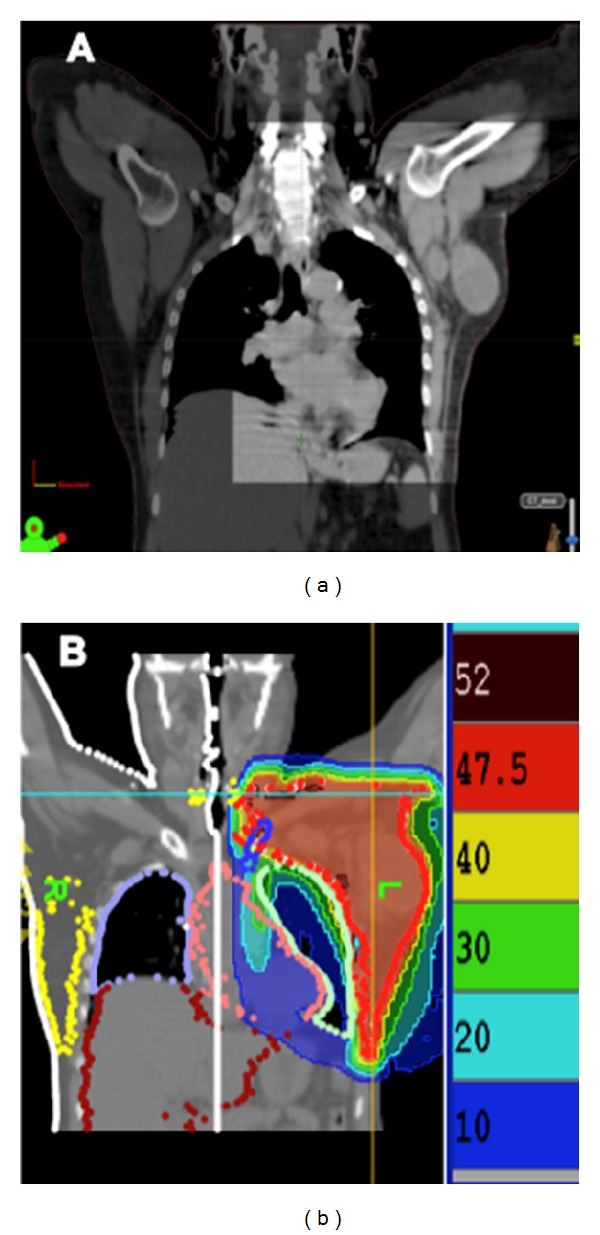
(a) Coronal view of planning CT scan. (b) Dose colorwash of helical tomotherapy (HT) treatment plan.
